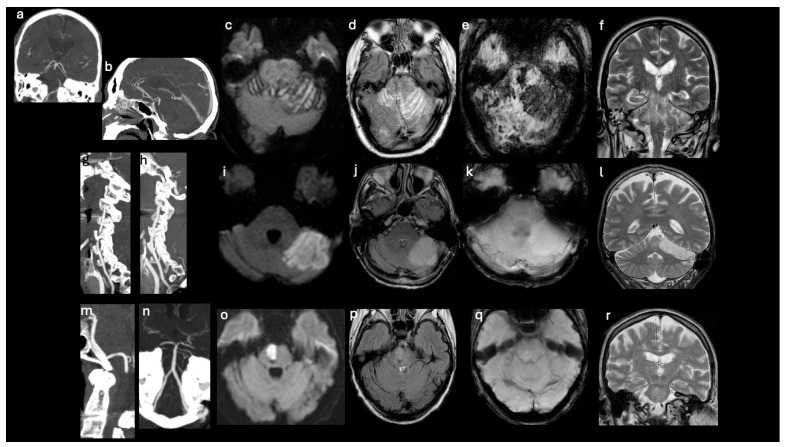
Topographic representation of ischemic lesions in patients with and without large vessel posterior circulation occlusion. CTA: (a,b,g,h,m,n); MRI: axial DWI (c,i,o); axial FLAIR (d,j,p); axial SWI (e,k,q); coronal T2 FSE (f,l,r). *Patient 1 (LVO)* (a–f). A 72-year-old man with a mid-basilar artery occlusion at CTA (a,b). Imaging shows an extensive, multiregional ischemic area involving the brainstem and cerebellar hemispheres characterized by T2 FSE, FLAIR, and DWI hyperintensity (consistent with cytotoxic edema) and SWI hypointensity (suggesting hemorrhagic infarction) (c–f). *Patients 2 (no-LVO) *(g–l). A 67-year-old man with a superior left cerebellar ischemic lesion, displaying T2 FSE, FLAIR, and DWI hyperintensity (indicative of cytotoxic edema) (i–l). No large vessel occlusion detected at CTA (g,h). *Patients 3 (no-LVO)* (m–r). A 66-year-old woman with an ischemic lesion on the right side of the pons, characterized by T2 FSE, FLAIR, and DWI hyperintensity (o–r). No large vessel occlusion detected at CTA (m,n). CTA, Computed Tomography Angiography; MRI, Magnetic Resonance Imaging; LVO, large vessel occlusion.
